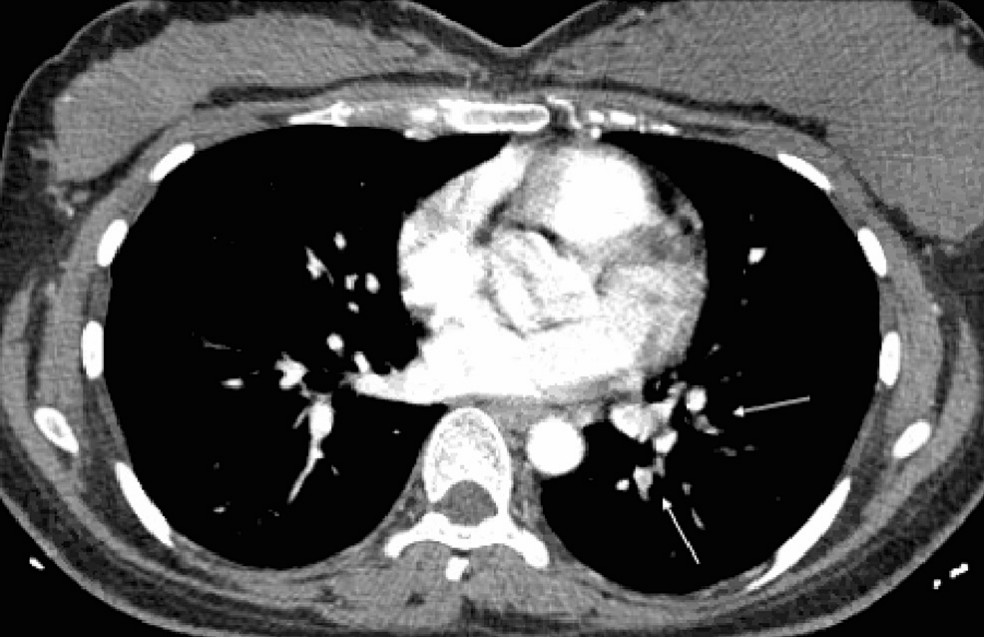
Axial view of CTPA revealing filling defects in the left lower lobe. CTPA: computed tomography pulmonary angiogram
